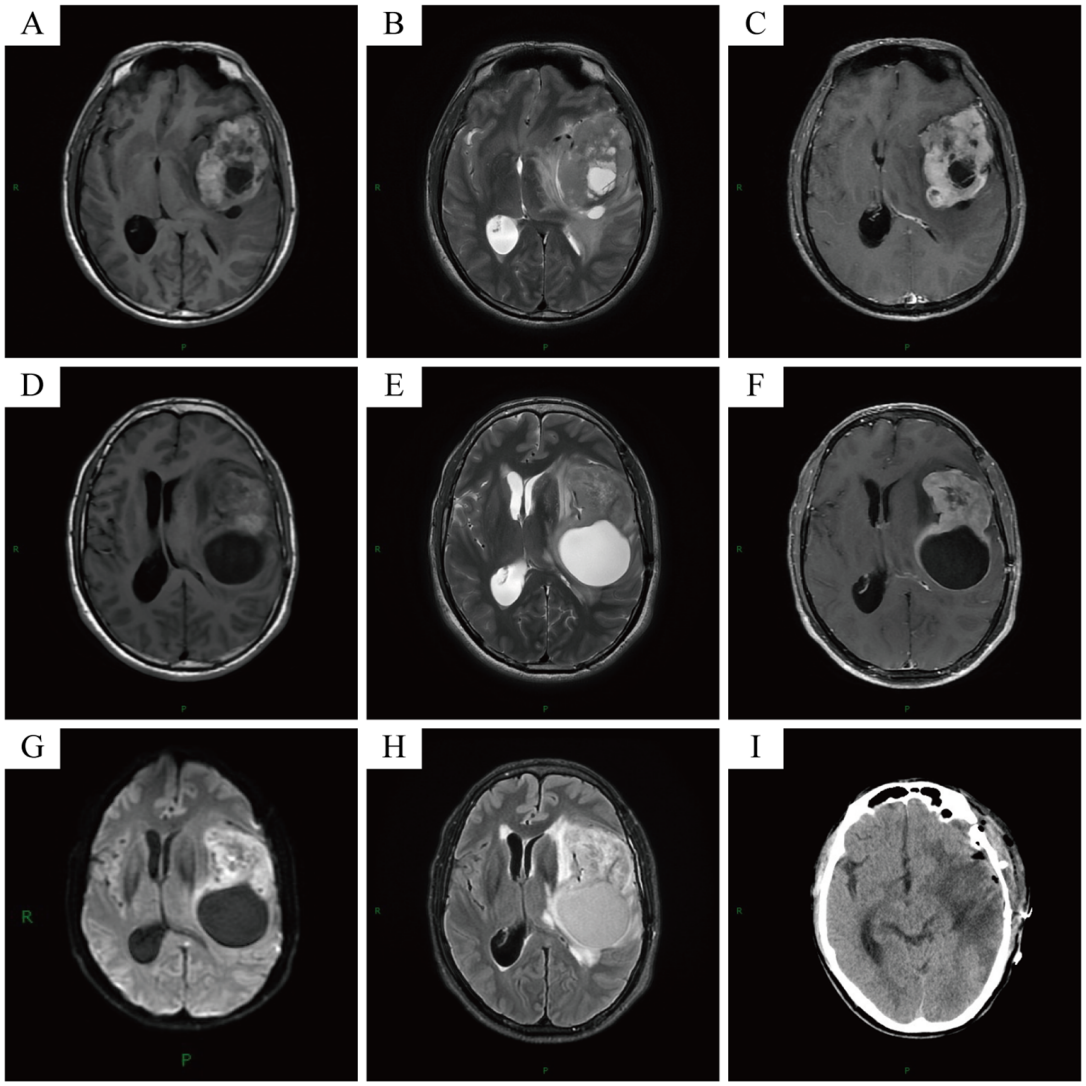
Initial MRI showed an irregular mixed signal mass in the patient’s left frontal lobe-insular lobe-basal ganglia region-temporal lobe, approximately 74×52×61mm in size (A-C). Pre-operative MRI showed an irregular mixed signal mass in the patient’s left temporal lobe, approximately 66×41×82mm in size (D-H). Post-operative CT showed post-operative changes in the patient’s left cerebral hemisphere (I).
